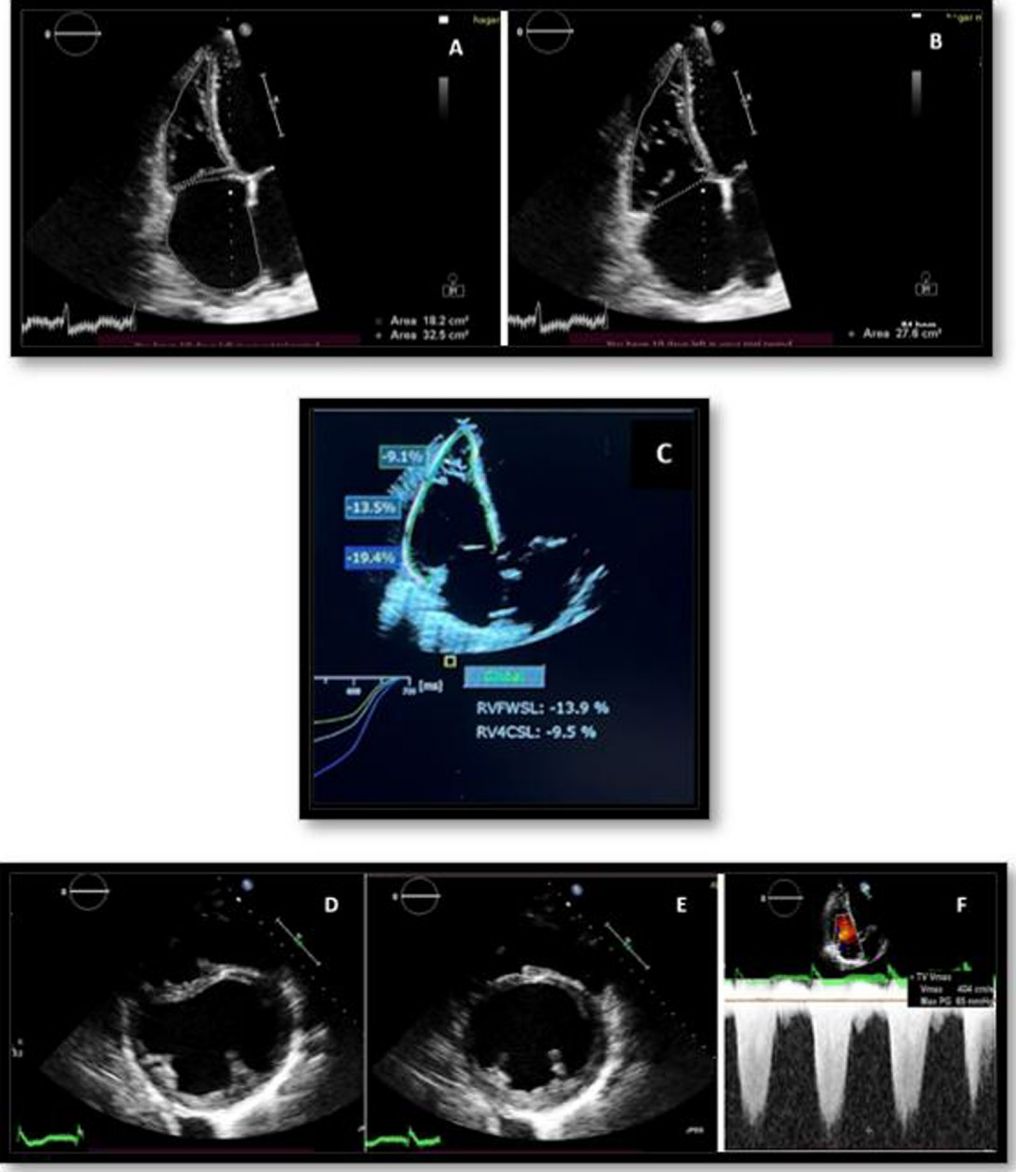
Echocardiographic findings in a patient with ischemic cardiomyopathy. A & B Dilated right ventricular end-systolic area (ESA) and end-diastolic area (EDA), as well as dilated right atrium (RA); & impaired RV fractional area change (FAC = 34%); C Impaired RV longitudinal strain; longitudinal RV free wall strain (RV FWS = − 13.9) and right ventricular four-chamber strain (RV4CSL = − 9.5%); D & E Parasternal short axis view, at papillary muscle level, showing septal shift towards the left ventricle, mainly during systole; F Continuous wave Doppler of the tricuspid valve showing high regurgitant jet velocity and estimated PASP = 65 mmHg
